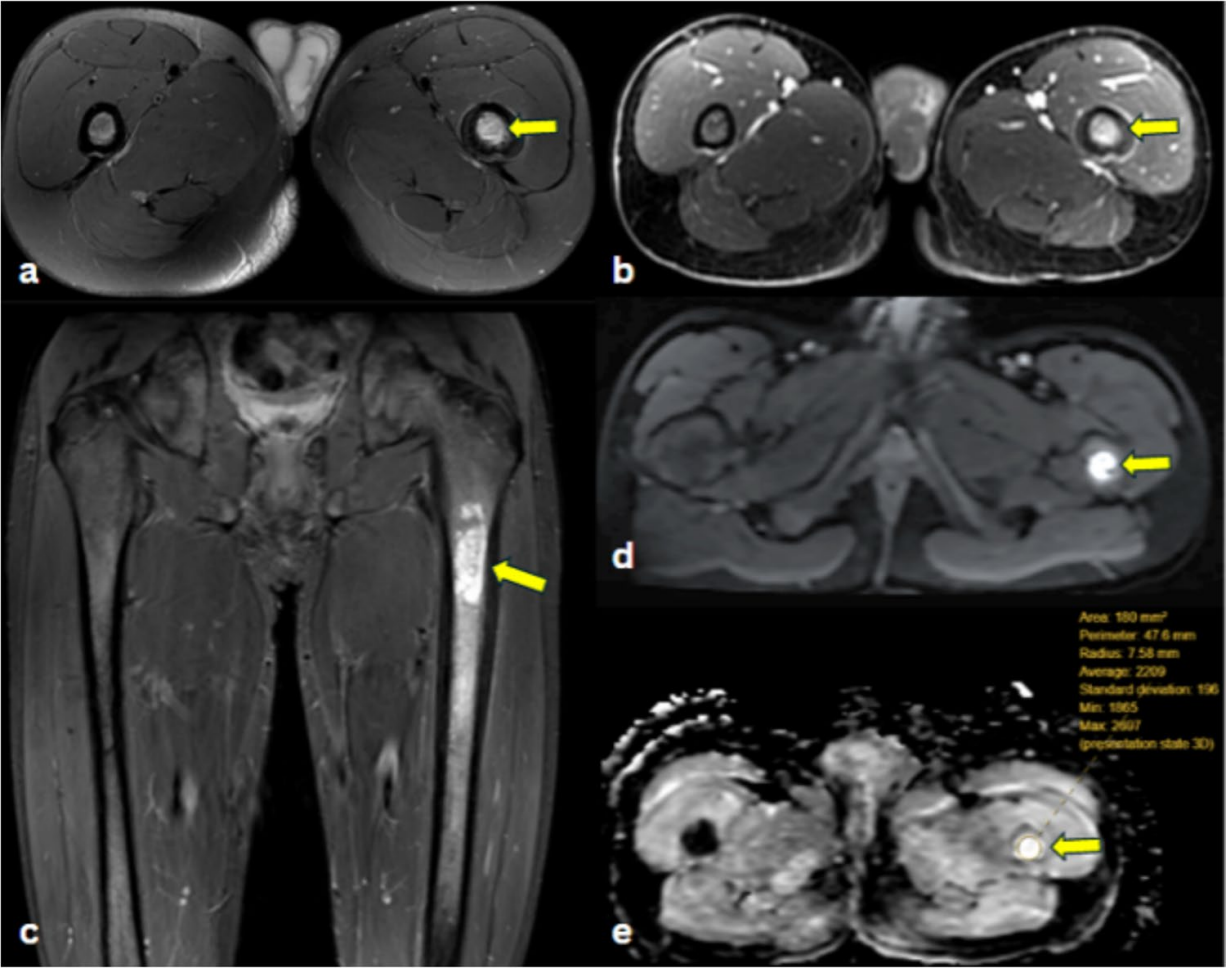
Enchondroma of left femur in a 15-year-old male. a Axial T2-fat-saturated image demonstrates an intramedullary hyperintense lesion in the mid-proximal left femur (arrow). b Axial post-contrast fat-suppressed T1-weighted image shows homogeneous contrast enhancement of the lesion (arrow). c Coronal short tau inversion recovery (STIR) MR image demonstrates a hyperintense subtrochanteric intramedullary multilobulated lesion in the mid-proximal left femur (arrow) with mild endosteal scalloping. d Axial DW-MRI reveals a hyperintense lesion (arrow) in the left proximal femur. e Corresponding ADC map shows hyperintense signal (arrow) of the lesion, indicating limited restricted diffusion, with ADCmean of 2.20 × 10^−3^ mm^2^/s, ADCmin of 1.86 × 10^−3^ mm^2^/s and ADCmax of 2.69 × 10^−3^ mm^2^/s
